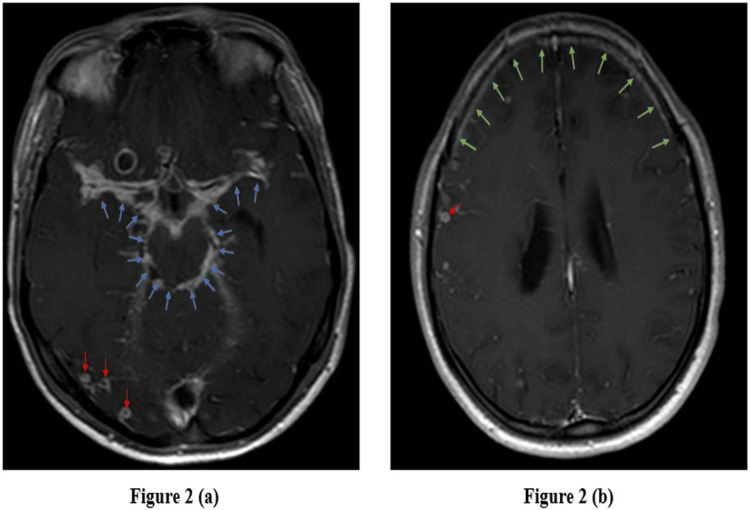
Axial post-gadolinium contrast T1-weighted MR images of the brain Axial post-gadolinium contrast T1-weighted MR images of the brain showing basal meningeal enhancement (marked by blue arrows) in (a) and diffuse meningeal enhancement (marked by green arrows) in (b) in a TBM patient. Also noted are multiple ring and nodular enhancing lesions (marked by red arrows) suggestive of tuberculomas. TBM: tuberculous meningitis
